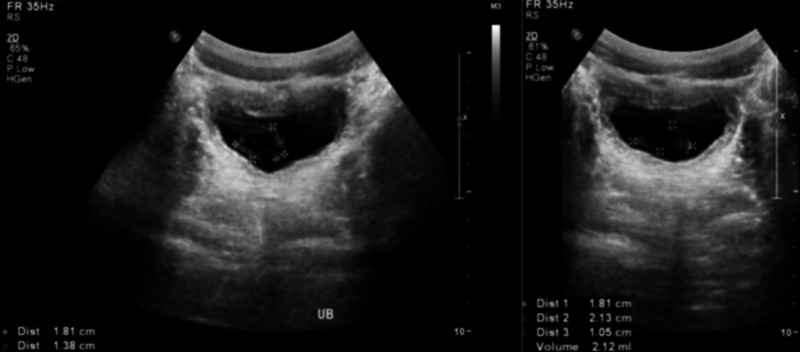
Renal ultrasound showing thickened urinary bladder wall
